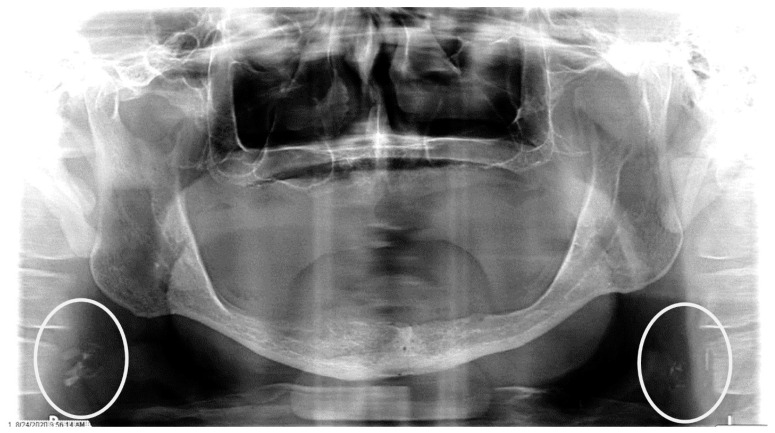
The panoramic radiograph of an 80-year-old male patient with a medical history of hyperlipidemia and diabetes mellitus showing bilateral carotid artery calcifications (CACs) during a comprehensive dental examination. The CACs are encircled by a white line.
